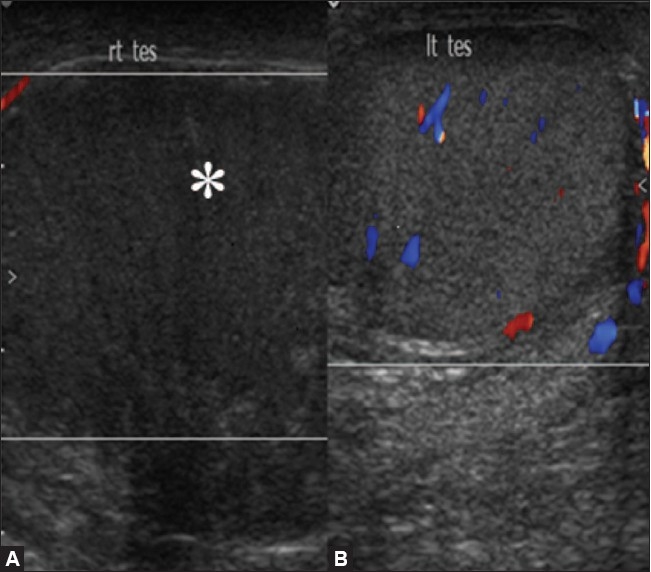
Torsion. Color Doppler shows a hypoechoic and enlarged right testis (arrow in A) showing absence of color flow with normal color flow and echo pattern in the left testis (B)
